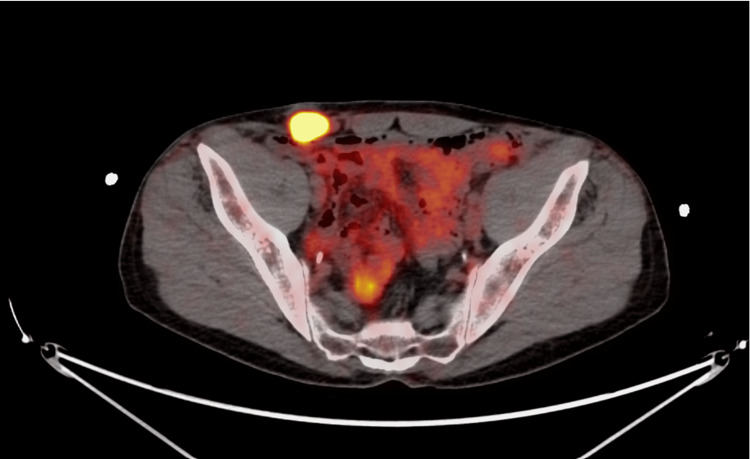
PET-CT confirming increased FDG activity at the subcutaneous mass FDG: Fluorodeoxyglucose
